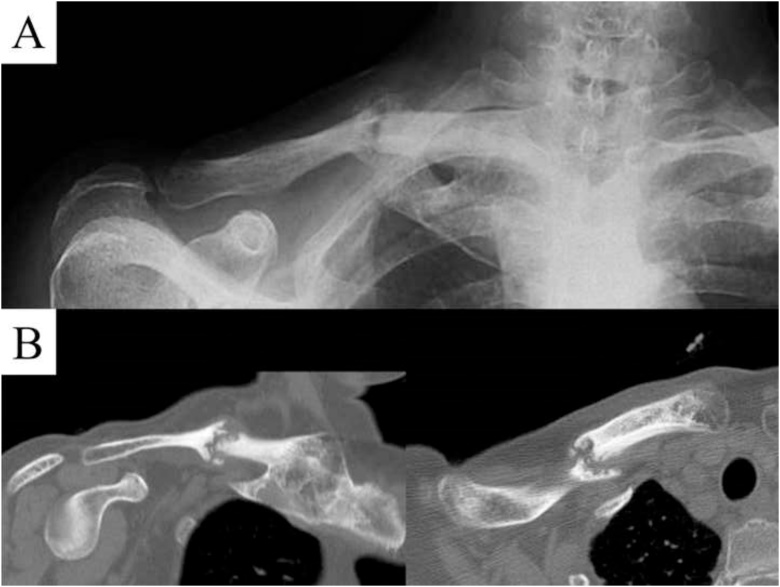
(A) Anteroposterior X-ray image of the clavicle at the time of admission. (B) Sagittal and axial computed tomography of the clavicle.
